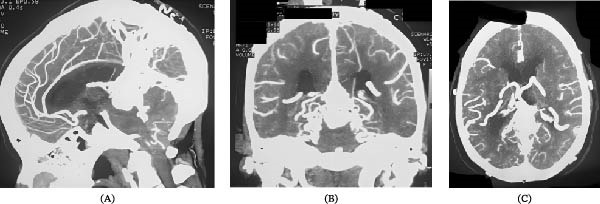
Contrast‐enhanced CT demonstrating a dilated midline venous pouch in the region of the vein of Galen draining into the straight sinus. (A) Sagittal: midline vascular structure draining into the straight sinus. (B) Coronal: dilated venous channel with multiple arterial feeders. (C) Axial: prominent tortuous vessels consistent with high‐flow arteriovenous shunting.
